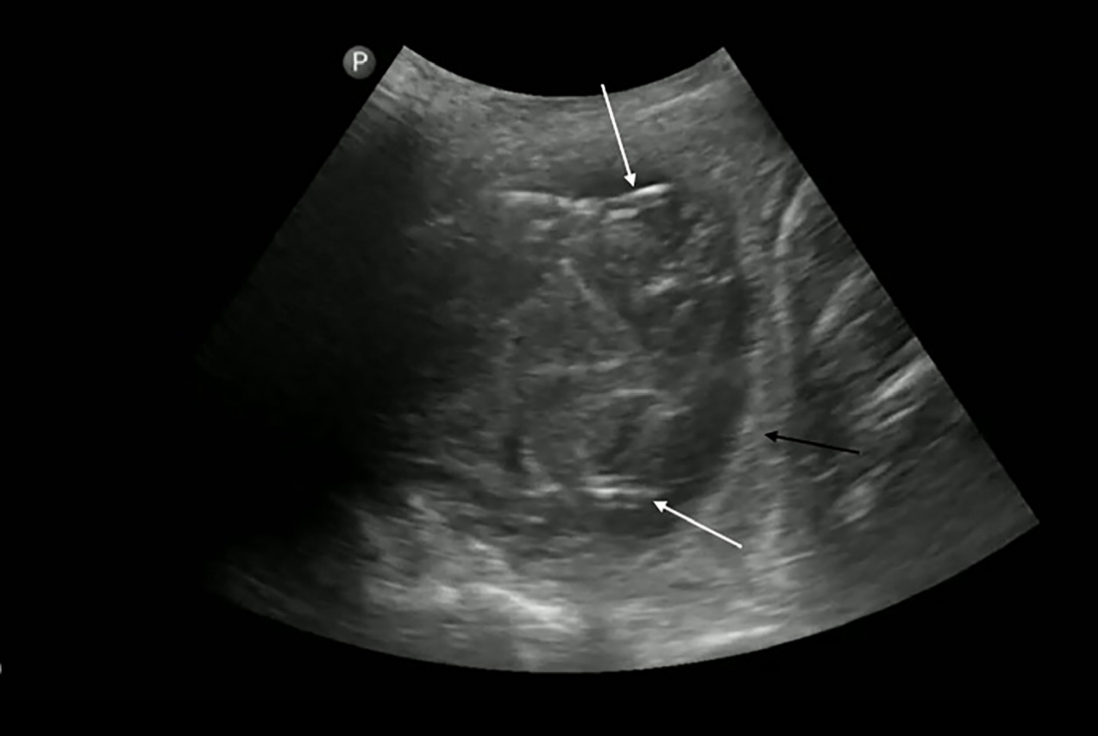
Point-of-care ultrasound of the left hemithorax showing the “honeycomb” appearance of a multivesicular cyst with double echogenic lines^6^ (white arrows) known as the “wall sign”^5^ and internal “serpentine” linear structures delineating the daughter cysts.^6^ This cyst occupies nearly the entire volume of the left hemithorax (black arrow indicates the diaphragm).
